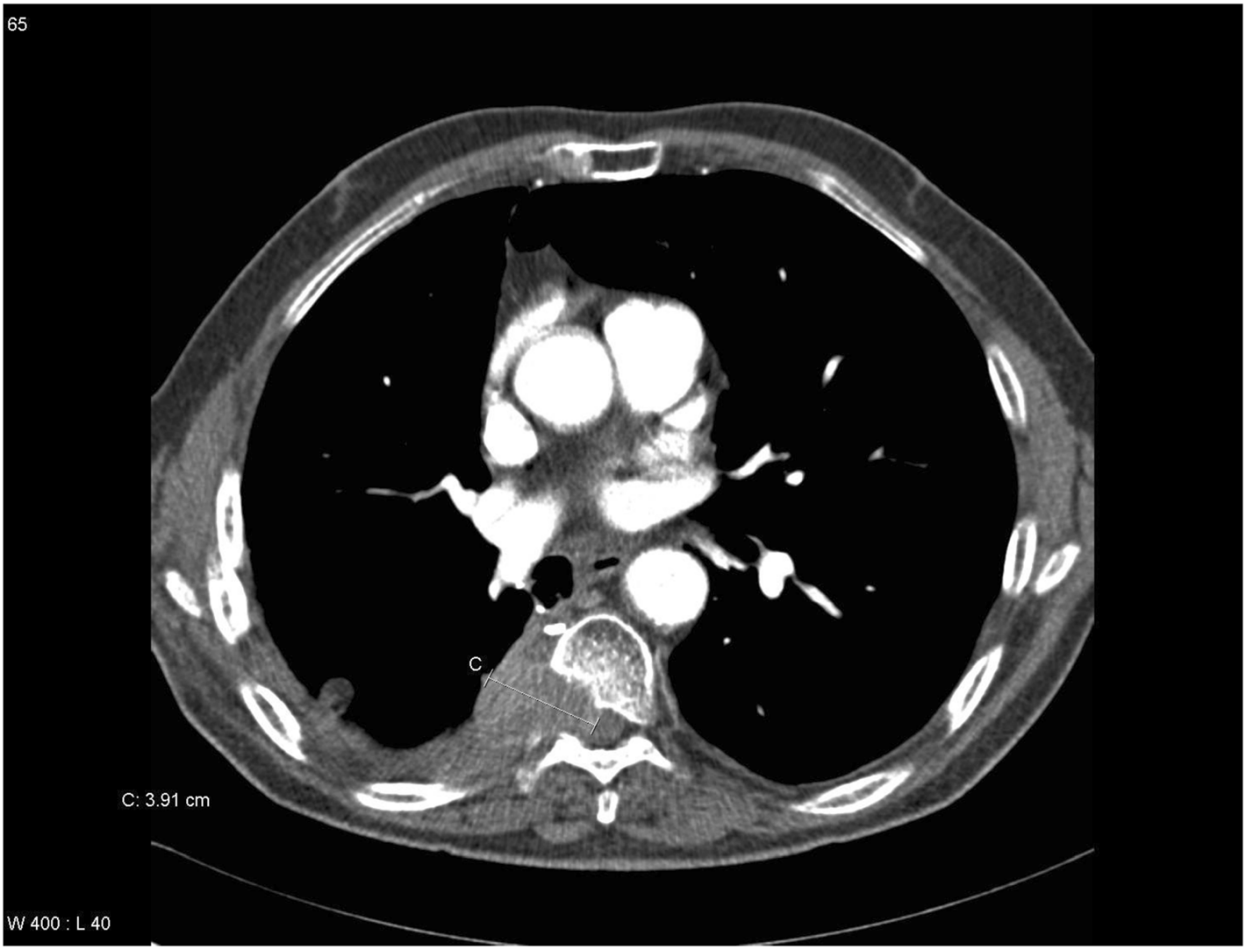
Computed tomography scan in a patient with squamous cell lung cancer (no EGFR mutation, known lung metastases) and uncomplicated bone pain (no neurological complaints), extra-osseous extension and spinal canal invasion present: treated with 3 Gy x10.
